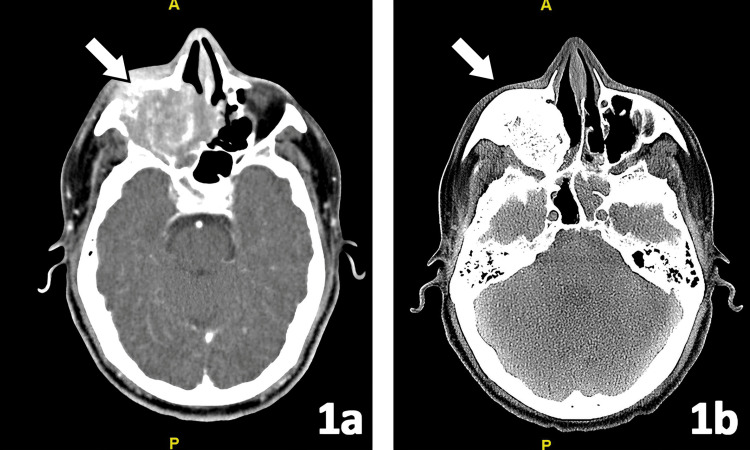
CT transverse section images (a, b) CT images of a patient before and after curative-intent treatment (site of primary lesion shown with an arrow mark)
